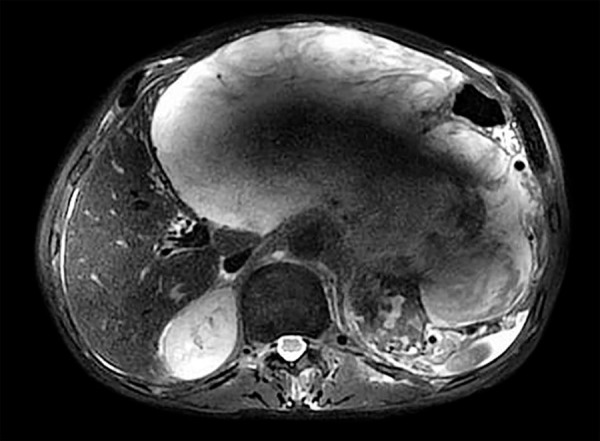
Inflammatory mass by MRI
